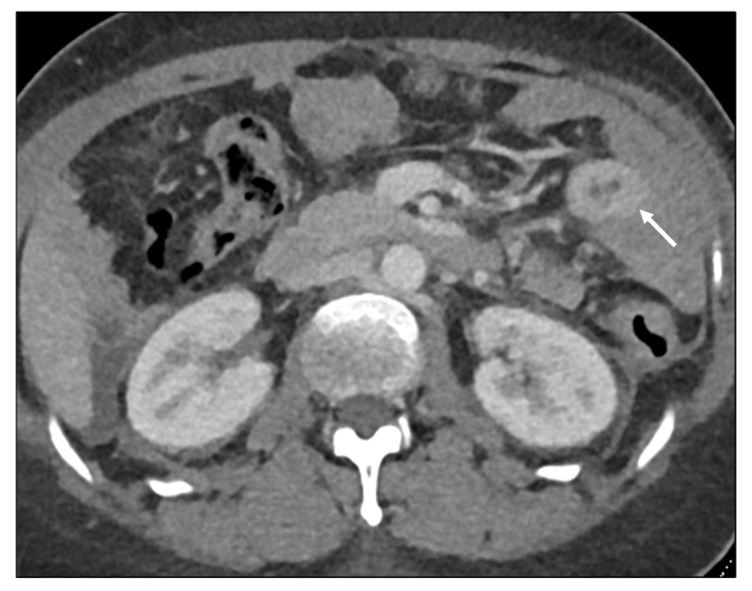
Homogeneous delayed enhancement. Axial contrast-enhanced CT. Metastasis to the descending colon (arrow) from undifferentiated gastric adenocarcinoma. Homogeneous enhancement at two minute-delayed phase. The layers are no longer discernible. This appearance correlates with the abundant desmoplastic reaction associated with linitis plastica.
